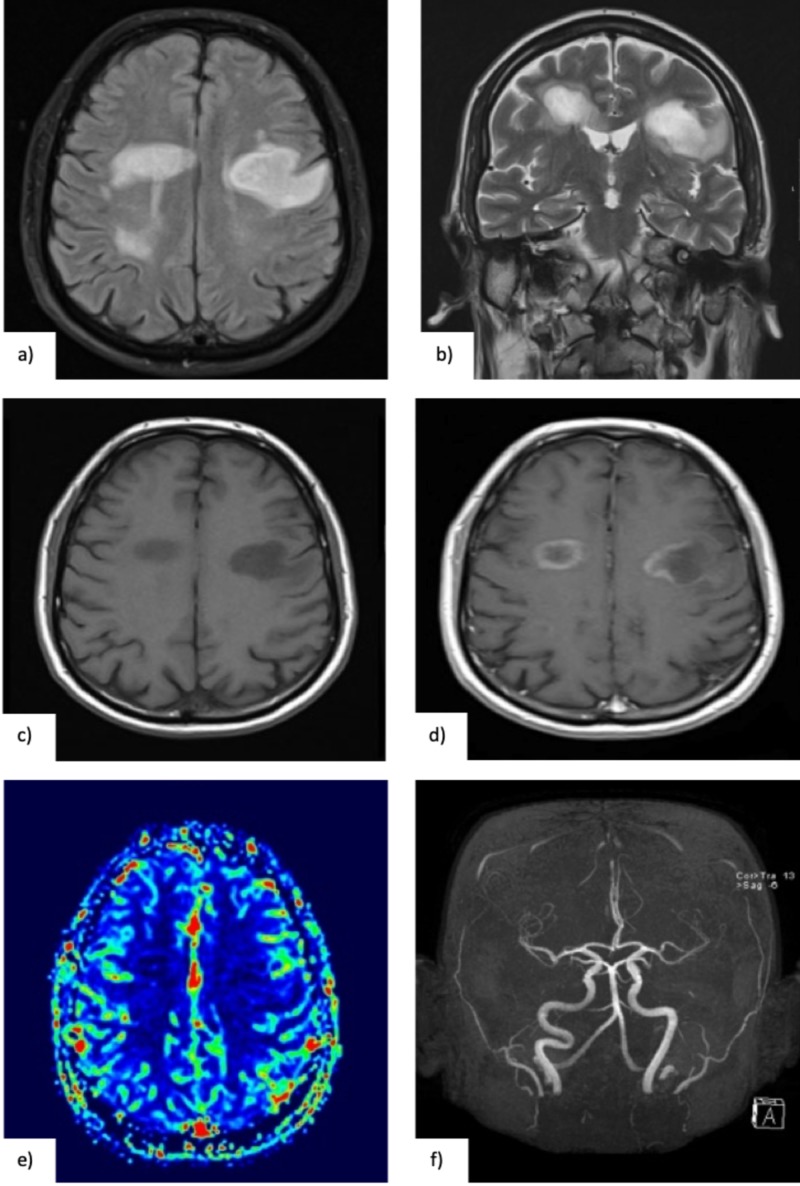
(a, b) Brain MRI (14-06-2017), showing white matter lesions, hyperintense in T2 FLAIR (axial) and T2 TSE (coronal) with surrounding oedema. (c) These lesions were hypointense in T1 (axial), and (d) presented open-ring enhancement after gadolinium injection. (e) Perfusion sequences revealed no increased perfusion in the mentioned lesions and (f) MRI angiogram was normal.
